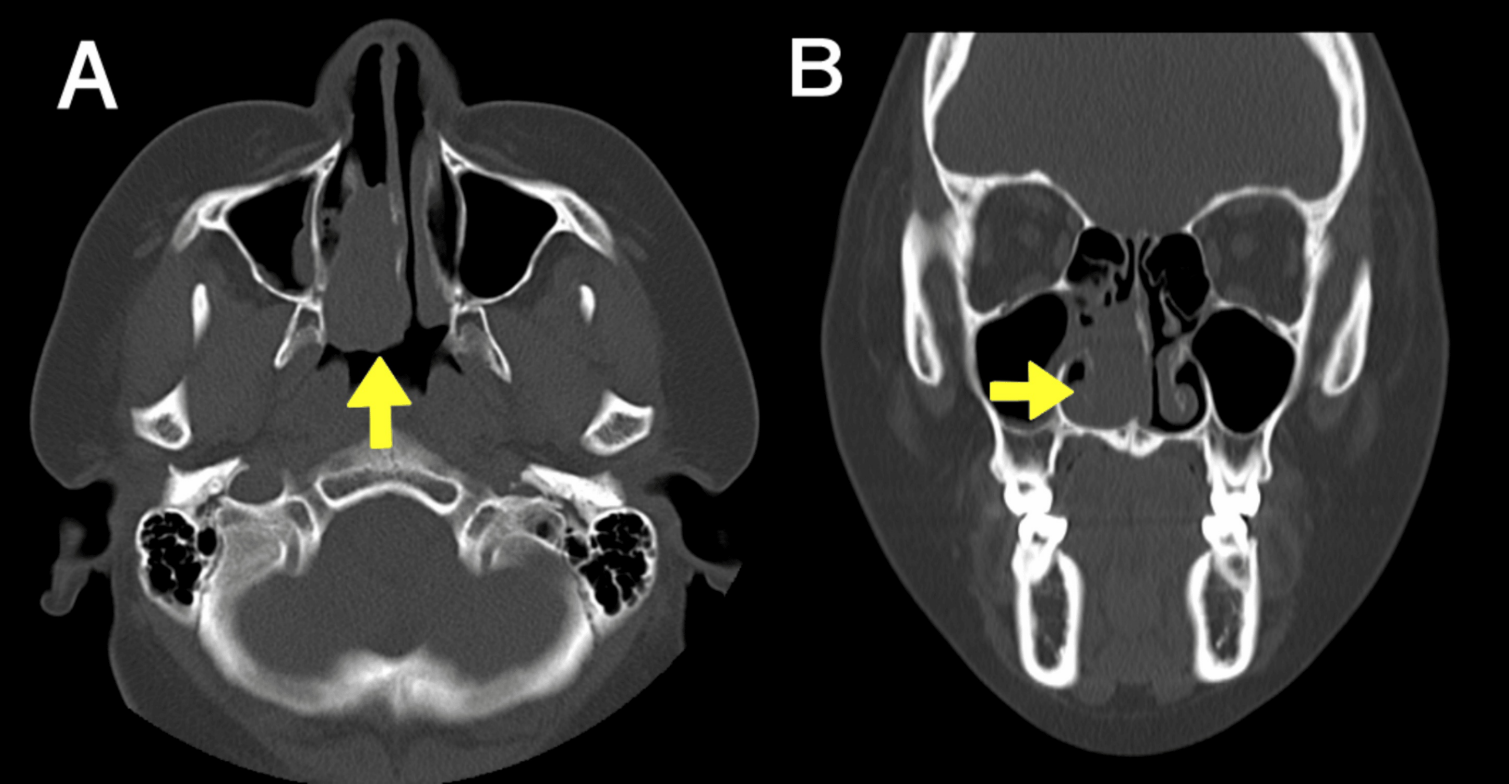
CT imaging before the initial surgical therapy Coronal (A) and axial (B) computed tomography (CT) images of the tumor before endoscopic surgery. The tumor was confined to the right nasal cavity with no bone invasion (arrow).
